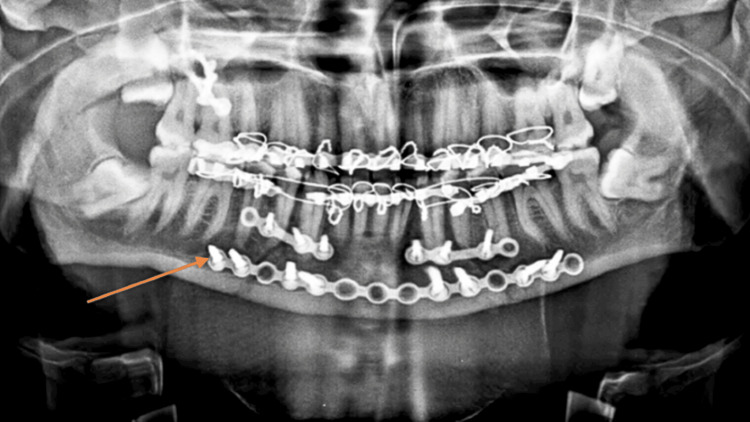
Post-operative orthopantomogram The orange arrow shows open reduction internal fixation of the bilateral parasymphysis and right zygomaticomaxillary buttress fracture and closed reduction of the right zygomatic arch fracture with intermaxillary fixation.
